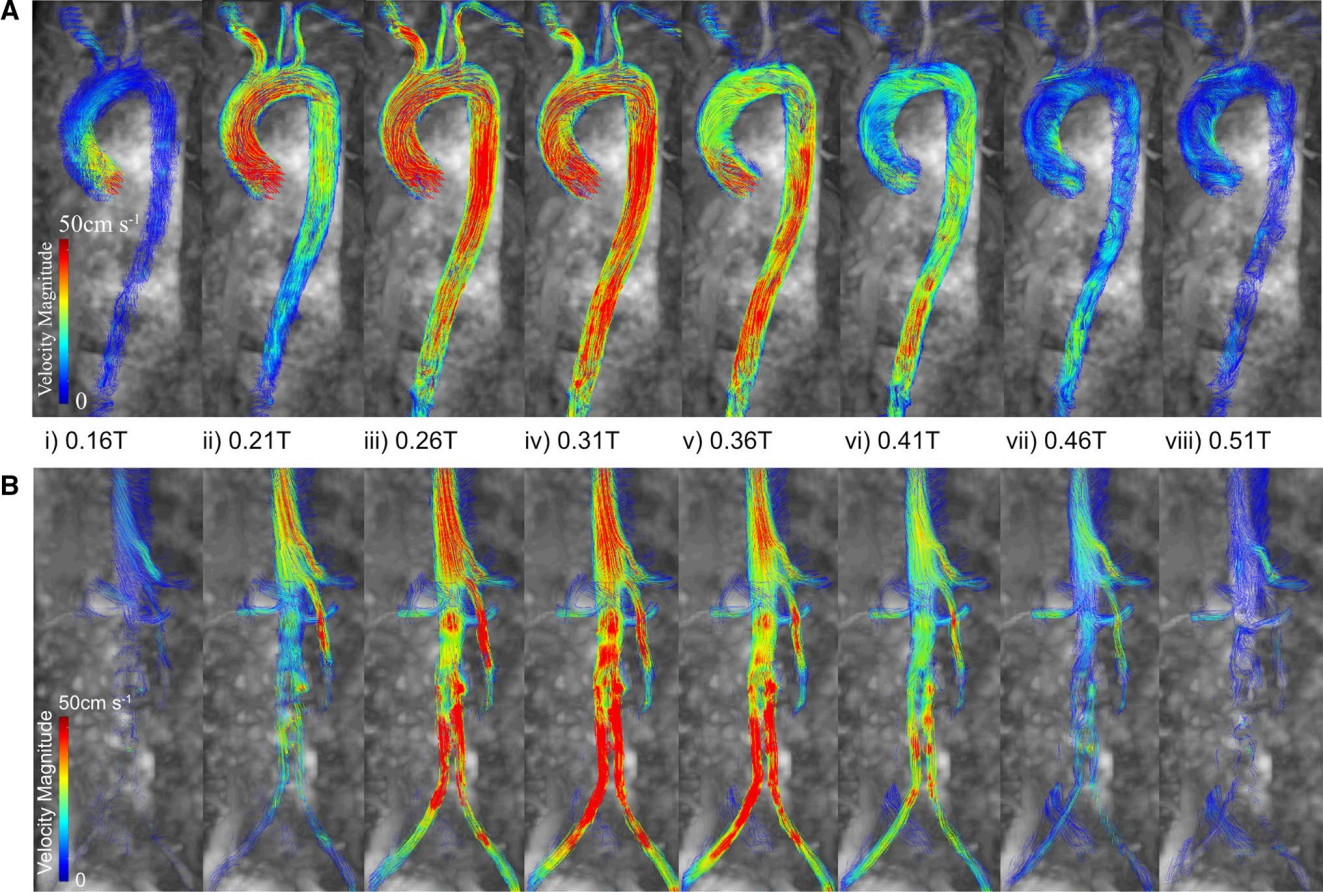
Velocity streamlines at consecutive time steps throughout A the thoracic aorta of a healthy volunteer, and B the abdominal aorta and common iliac arteries of patient 1 with an Anaconda™ stent-graft. This was obtained from analysis of 4D Flow-MRI data on circle cardiovascular imaging software, cvi42®. All images are shown between a velocity scale of 0–50 cm s^−1^ at time points throughout the cardiac cycle, where T is the cardiac period (0.21 T: Systolic acceleration (SA); 0.26 T: Peak systole (PS); 0.36 T: Systolic deceleration (SD))
